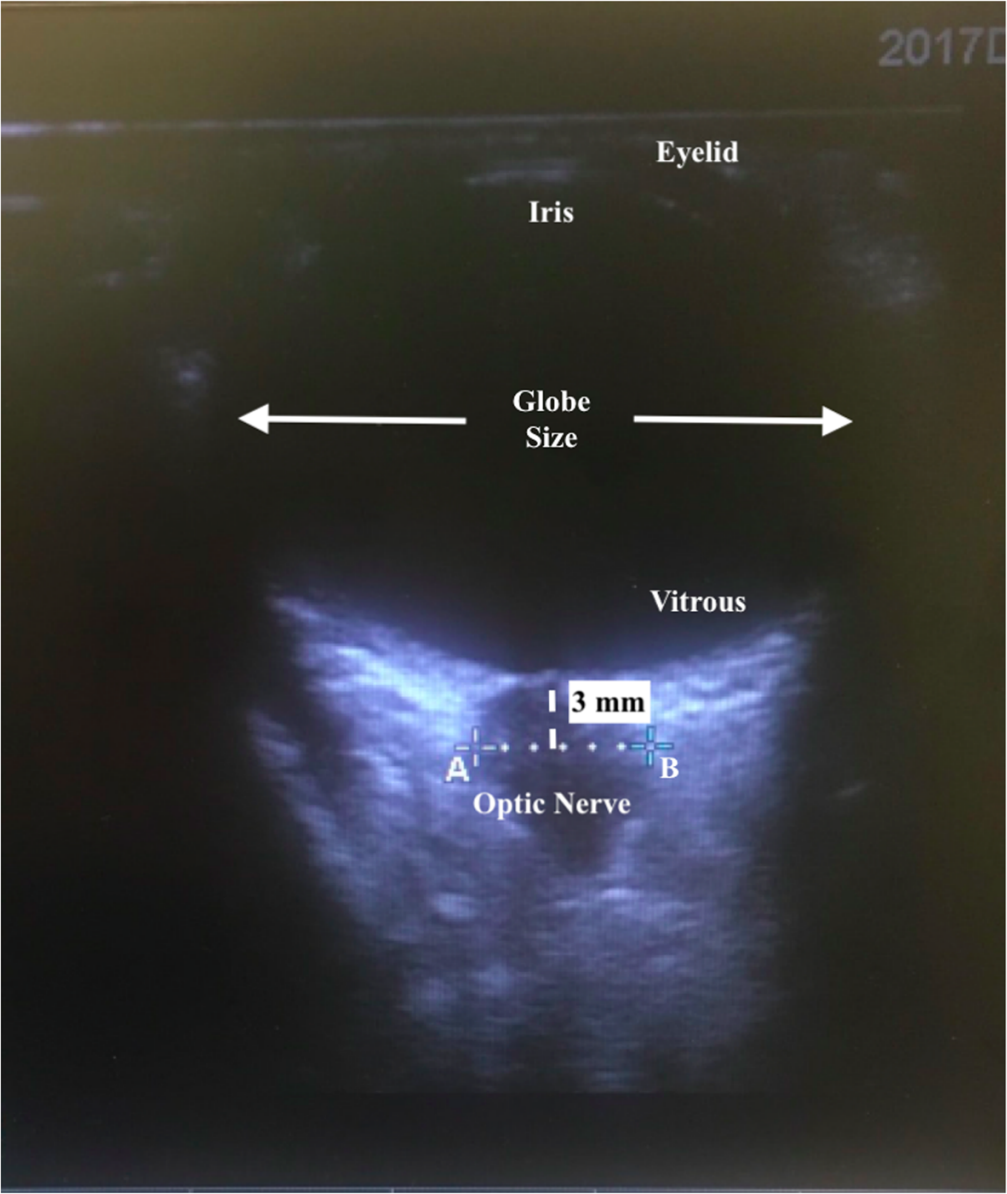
The ultrasonographic view of the axial axis of optic nerve. Optic nerve sheath diameter was measured between the A and B points at 3 mm posterior to the globe
